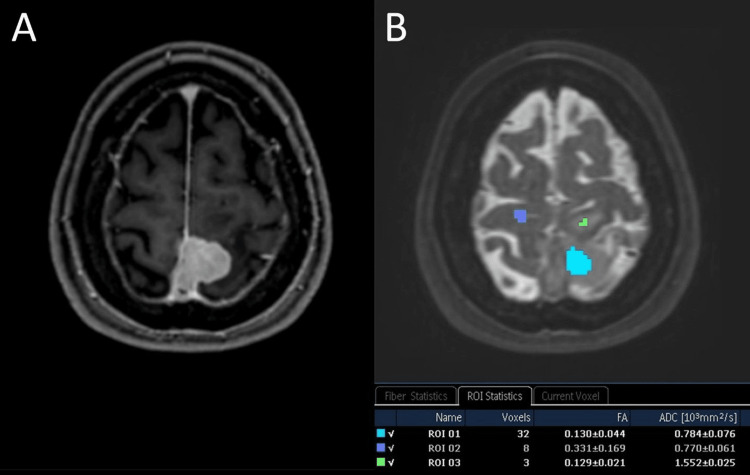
Axial post-contrast T1-weighted image at the level of the intracranial tumor mass (A). Corresponding DTI image with ROIs for the calculation of the FA and ADC values (B): light blue – tumor mass, green – white matter edema, purple – normal gray matter.
